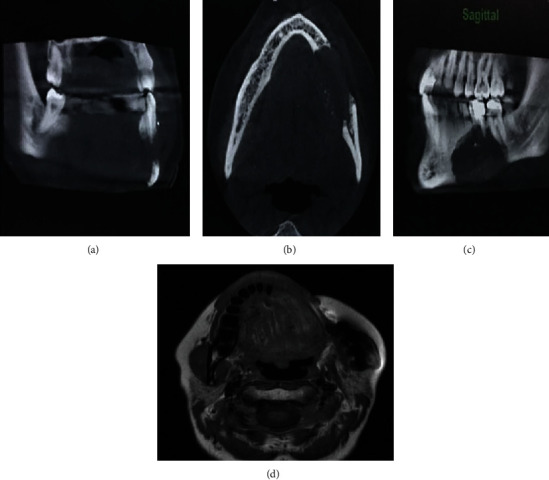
(a–c) Coronal, axial, and sagittal CBCT views showing severe buccal and lingual cortical plate perforation and IAC involvement by the tumor. The small radiopaque foci marked with arrows (b, c). (d) T1-weighted MRI showing left hemimandibular excision with its ipsilateral masseter muscle resection from the left lateral incisor area.
